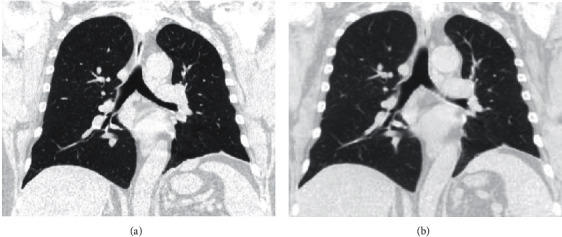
Comparison of coronal images from noncontrast, high-resolution chest computed tomography (CT) before and after COVID-19 pneumonia. (a) demonstrates a coronal slice of the patient's high-resolution chest CT 1.5 years prior to presentation. (b) is a follow-up chest CT 3 months after the patient's recovery from COVID-19 that shows absence of residual pneumonitis or new fibrosis.
